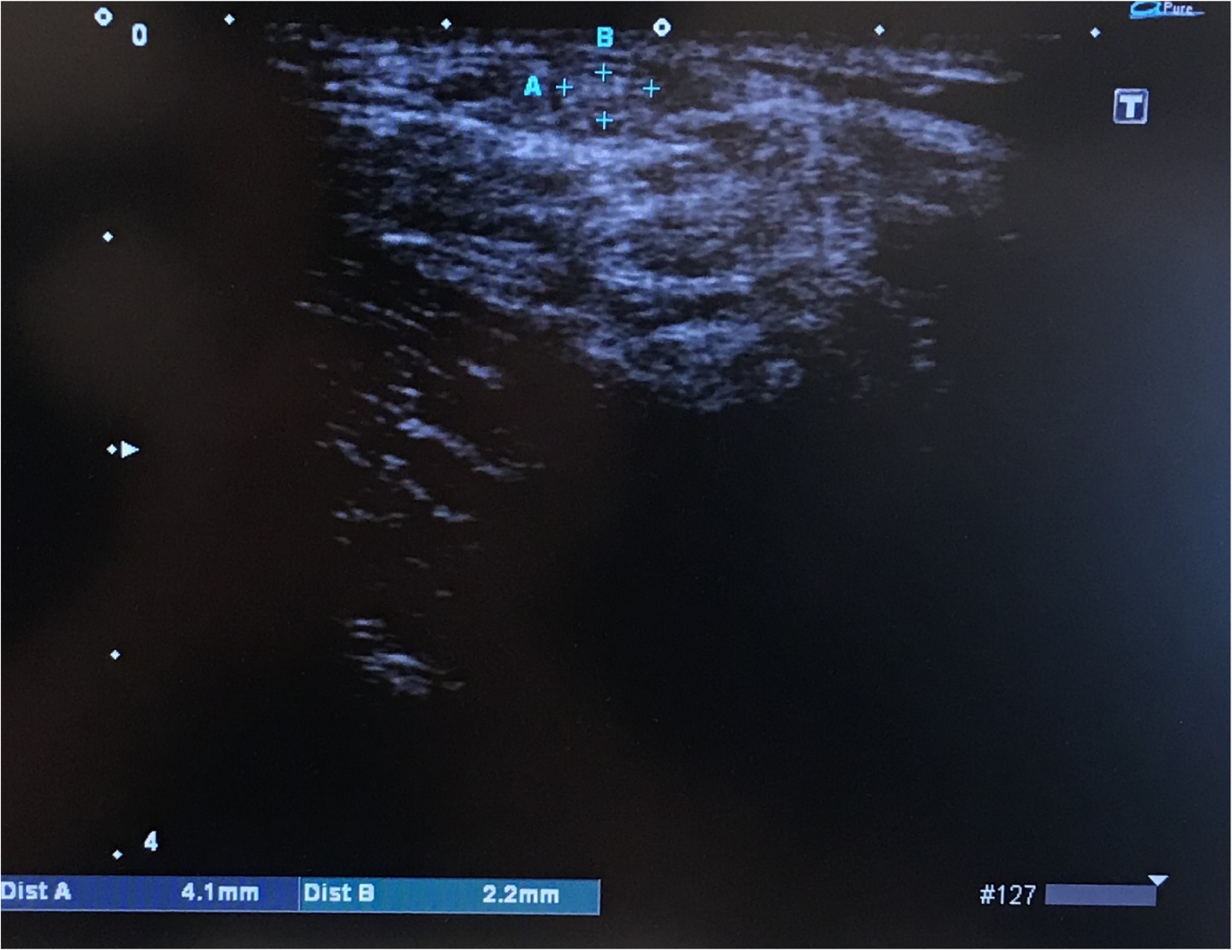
Ultrasonography images of the short-axis view. The diameter (a) and breadth (b) of the semitendinosus tendon
